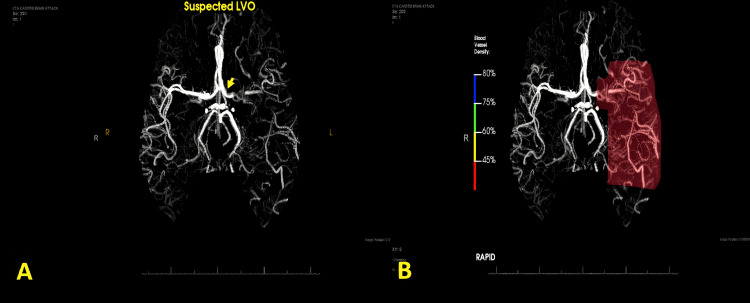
In image A on the left CT angiography reconstruction imaging reveals filling defects in the (yellow arrow) left terminal ICA, M1 and M2 segments of the MCA compatible with large vessel occlusion/thrombosis. In image B on the right, blood vessel density in left MCA territory was estimated by AI vessel density mapping technology to be severely reduced, greater than 55%. ICA, internal carotid artery; MCA, middle cerebral artery; AI, artificial intelligence
